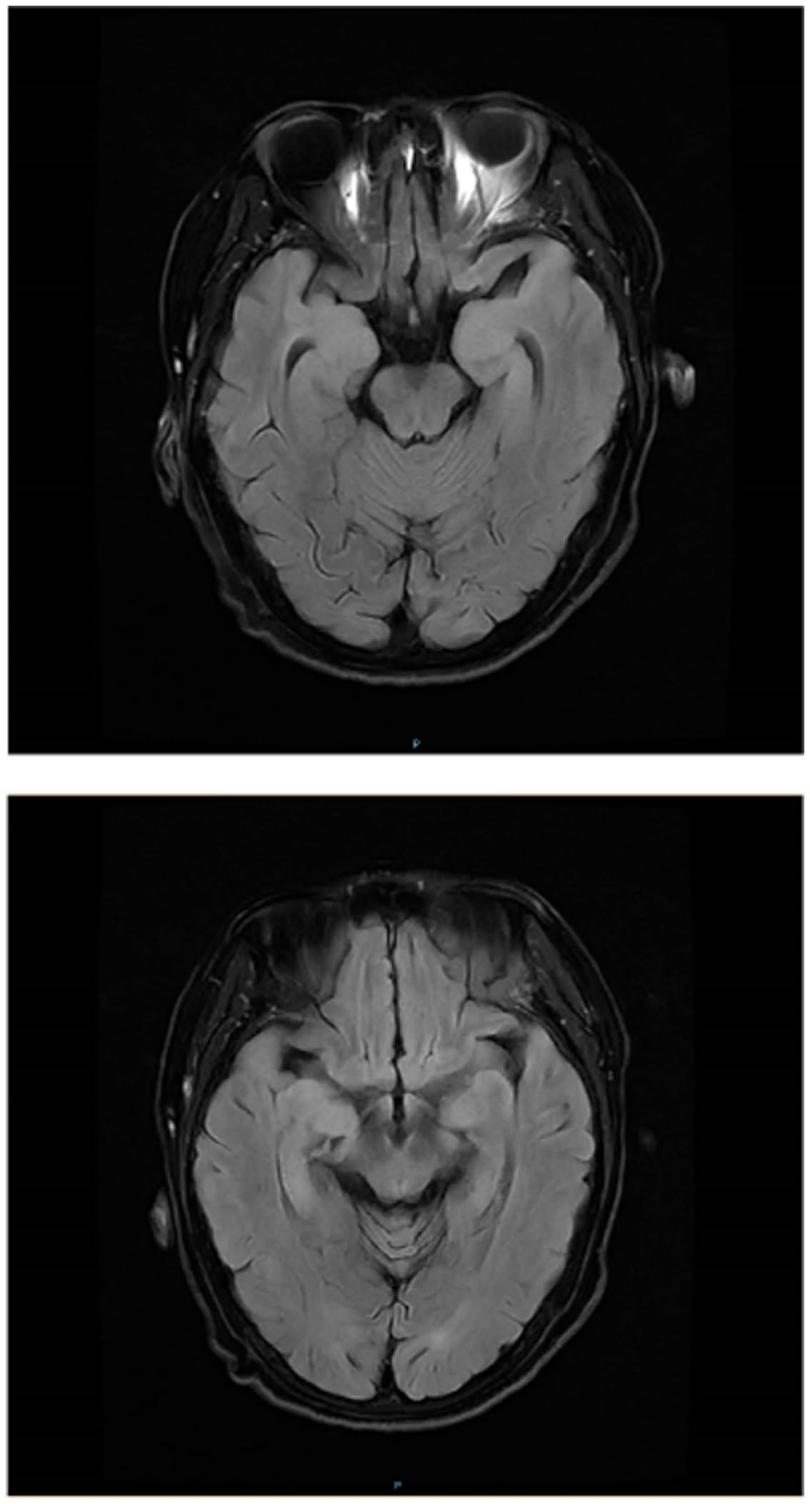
The above depict the head magnetic resonance imaging (MRI) findings of patients with confirmed anti-LGI1 encephalitis. These images demonstrate T2-FLAIR hyperintensities in the bilateral amygdala, hippocampus, and insular lobe.
